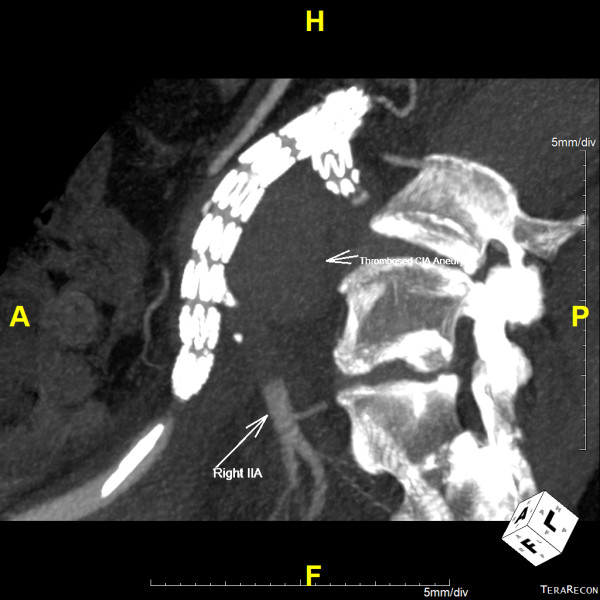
Maximum intensity projection reconstruction of a postoperative computed tomography angiogram, demonstrating thrombus in the right common iliac artery aneurysm sac with no evidence of endoleak and retrograde filling of the right internal iliac artery stump.
